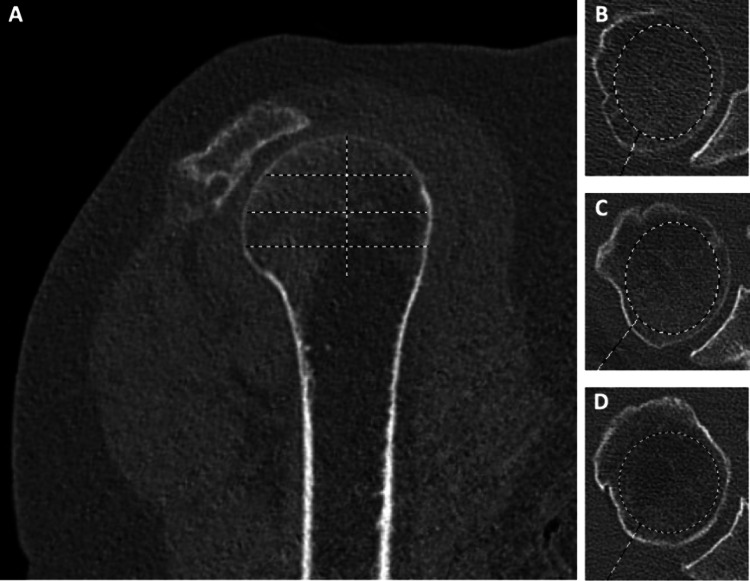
Example measurement of bone mineral density of the humeral head by CT method. A line is drawn on a sagittal cut from the superior humeral head to the surgical neck. This line is divided into quarters, with the dividing lines providing 3 axial slices. An ellipse is drawn on each identified axial cut to measure Houndsfield units (HU) excluding all cortical bone. The average of the 3 measurements is taken as the final value [24]
